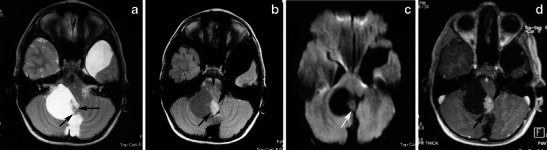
A large, cystic CPA in a 7-year-old boy. (a) An axial T2-weighted image shows a hyperintense mass of the right cerebellar hemisphere with a less intense soft-tissue nodule along its medial margin and without surrounding oedema (arrows). Note the arachnoid cyst in the left middle cranial fossa. b On an axial FLAIR image, the cystic component shows low signal intensity that is higher than that of the CSF, and the soft tissue nodule is homogeneous and slightly hyperintense (arrows). c On a diffusion-weighted image, the mural nodule appears isointense (arrow). d An axial, contrast-enhanced, T1-weighted image demonstrates intense enhancement of the mural nodule (arrows)
